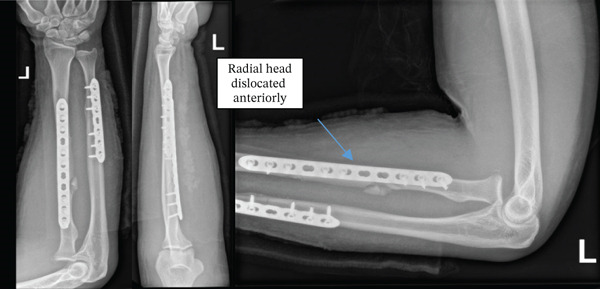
AP and lateral radiographs after application of the backslab.
